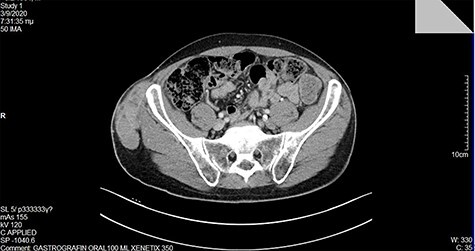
A large lipomatous mass detected in the MRI.
